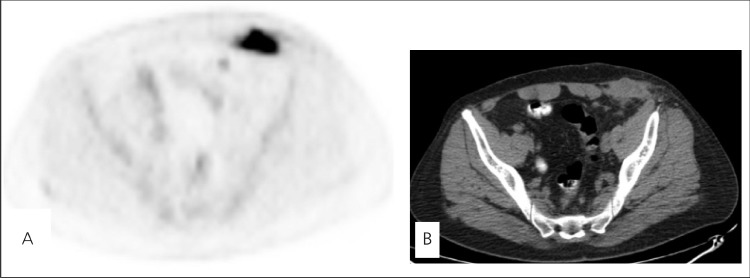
A, B. Axial PET image of the pelvic region shows an intenseFDG uptake at left anterior abdominal wall corresponding to repairedhernia region with surgical mesh implant, with a maximum standardizeduptake value (SUV) of 17.5. Axial CT image demonstrates softtissue thickening including a hypodense area
